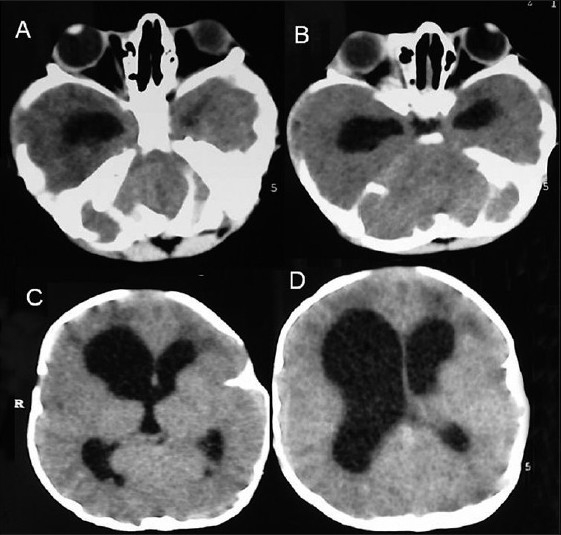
Case 2: (A–D) Computed tomography scan showing an asymmetrical ventricular dilatation (right lateral ventricle more than the left one). The fourth ventricle was not visualized. There is bilateral proptosis with shallow orbits
